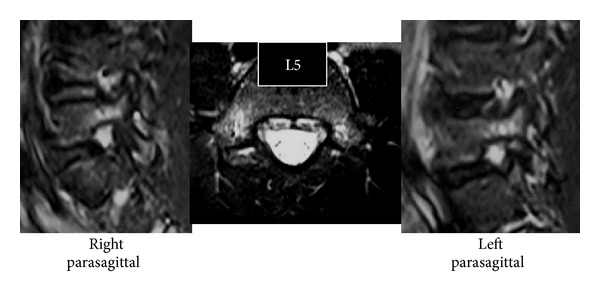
STIR-MRI of Case 3. Note the high signal changes of the pedicle, indicating marrow edema. This is a sign that the adjacent pars would have an early stage stress fracture of the pars interarticularis.
